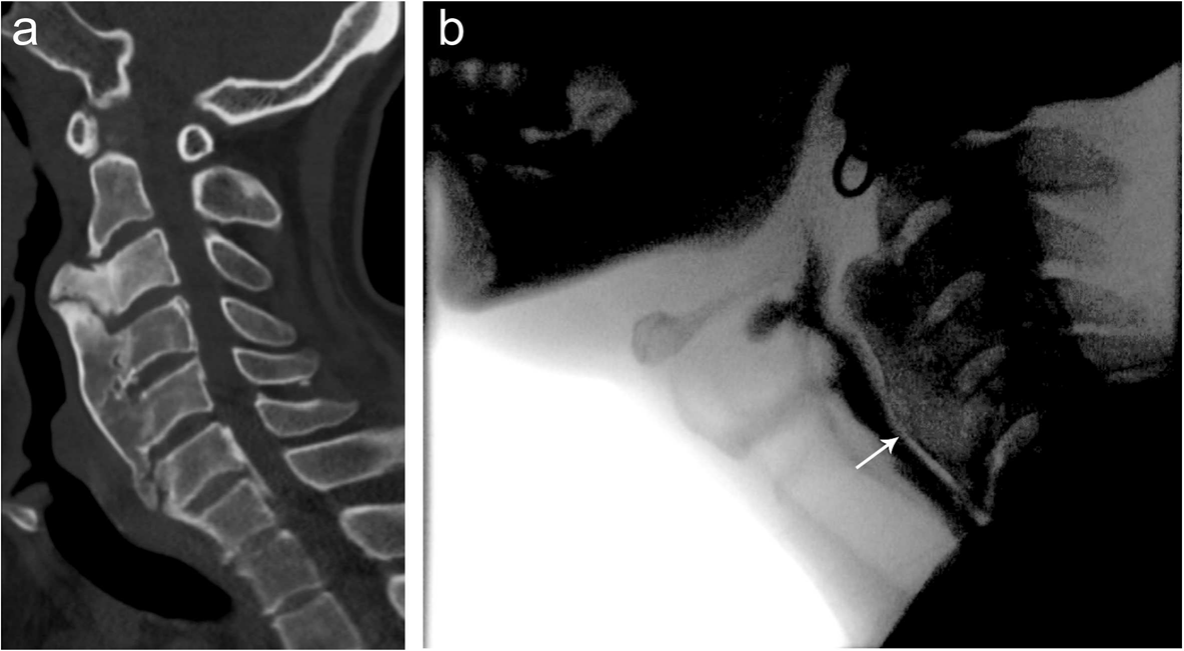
Diffuse idiopathic skeletal hyperostosis. Sagittal (a) noncontrast CT image of the cervical spine demonstrating large anteriorly flowing osteophytes extending from the C3–C7 levels without significant disc height loss consistent with the diagnosis of diffuse idiopathic skeletal hyperostosis (DISH). Lateral (b) fluoroscopy during a pharyngogram demonstrates posterior compression (arrow) of the cervical esophagus during swallowing
